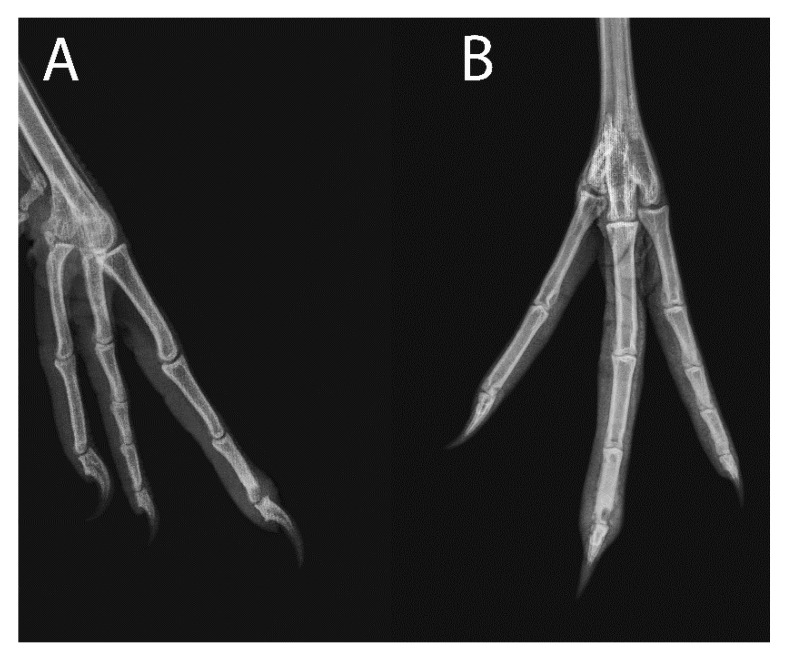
(A) Lateral and (B) dorsoplantar radiographs of the right foot of Case 2, a 31-year-old male Siberian crane (*Leucogeranus leucogeranus*) with probable osteomyelitis of the third digit of the right foot at time of presentation.
